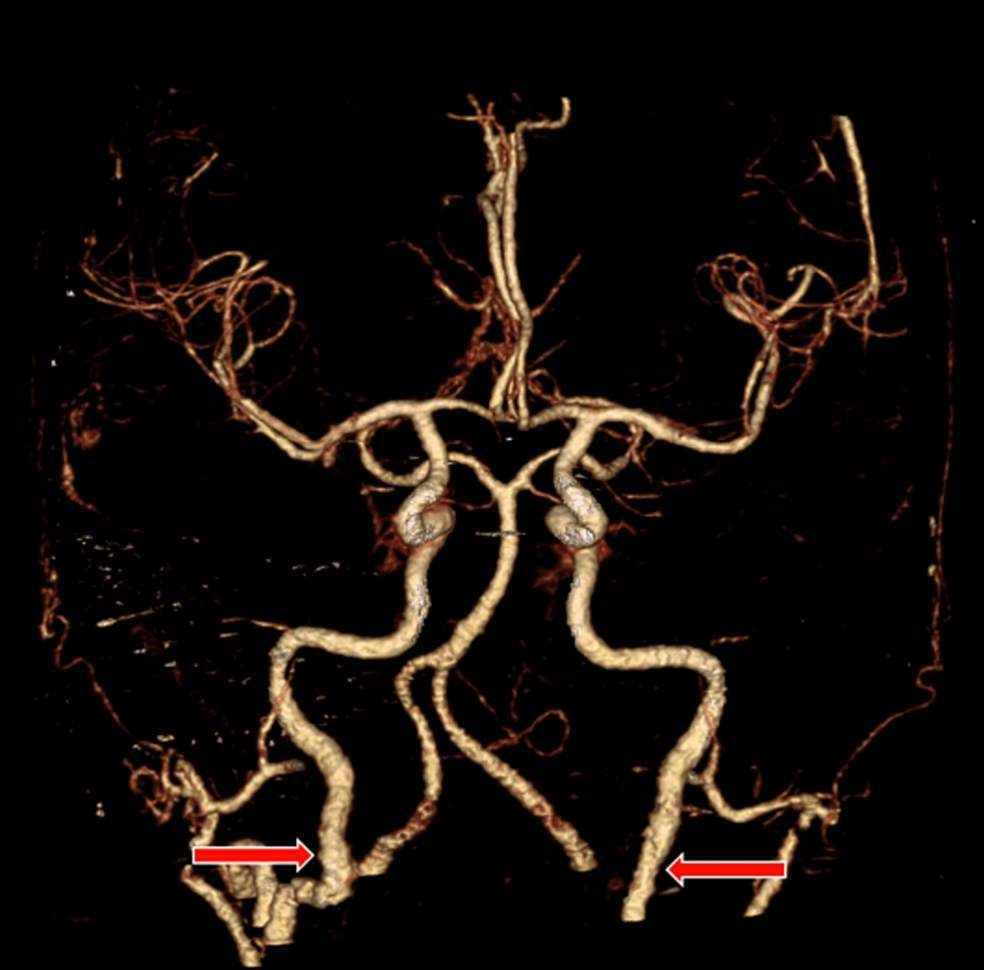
3D Volume rendered CT angiogram The angiogram demonstrates no intracranial arterial abnormality. Mild contour irregularities of both cervical internal carotid arteries are partially seen (red arrows).
